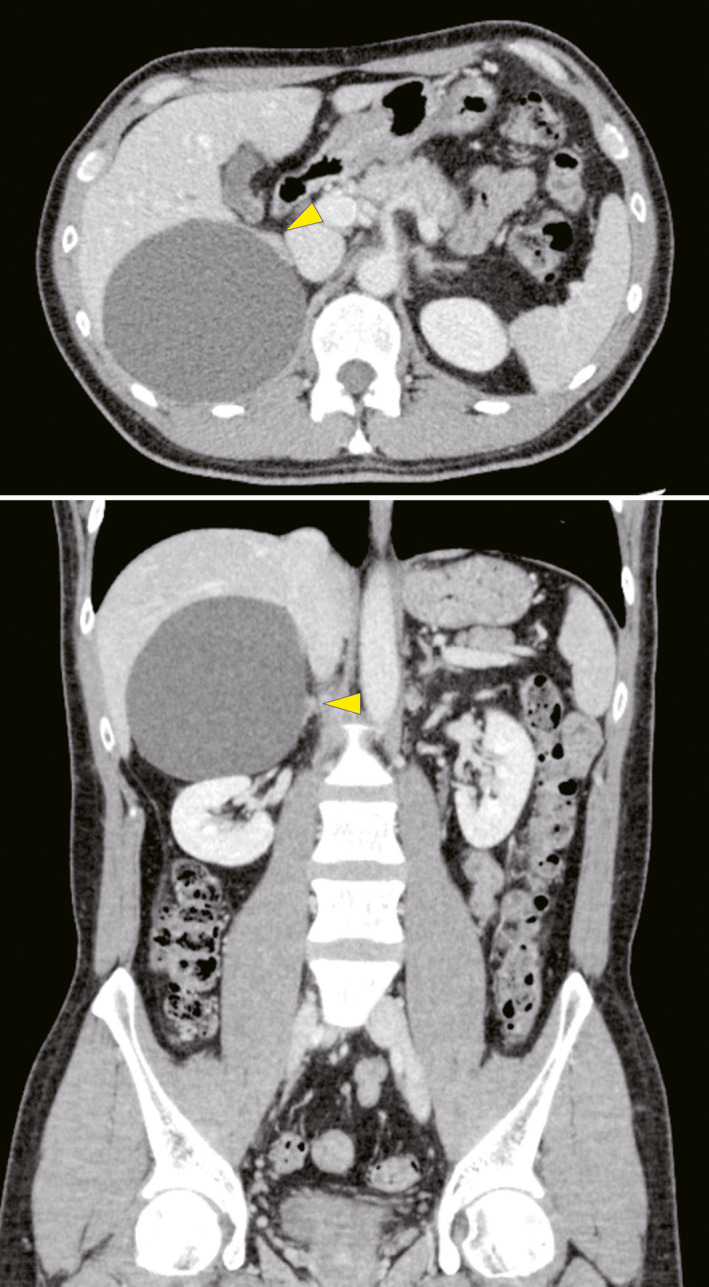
Preoperative CT image of the patient. Transverse (top) and coronal plane (bottom). A 10‐cm adrenal cyst and a normal adrenal gland (arrowhead) compressed by the cyst were seen.
